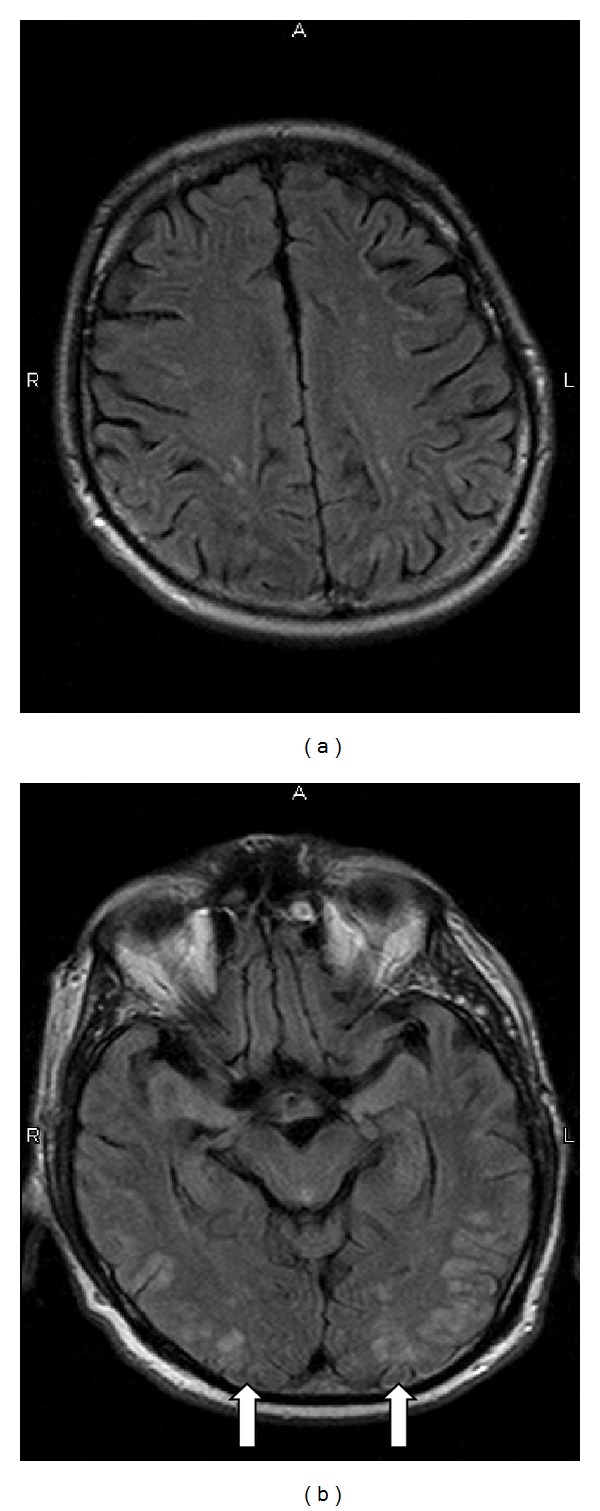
FLAIR AXIAL MRI images (TR 4140, TE 117) of the initial admission demonstrating multiple small bilateral cortical hyperintensities especially involving the occipital lobes (arrows). There was no evidence of mass effect or contrast enhancement on postcontrast images (not shown).
